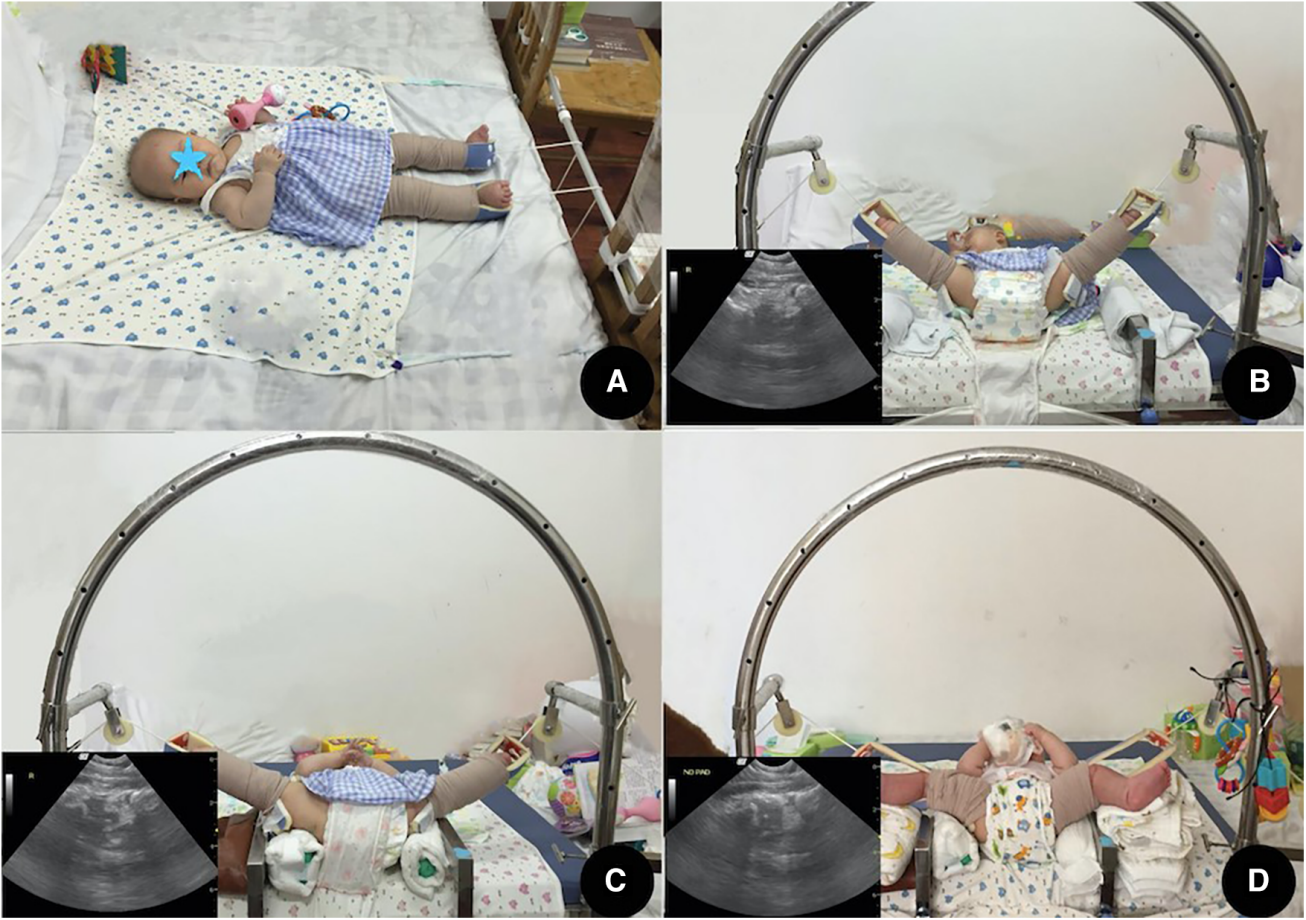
Two-stage traction. Stage 1: (A) horizontal traction at home. Stage 2: (B–D) ultrasound-guided vertical traction in the hospital. (B) Day 4, abduction was increased to 50°, and anterior hip ultrasound viewed the posterior dislocated femoral head. (C) Day 7, after using padding and adding the traction load, the femoral head was reduced to the acetabulum, but some distance between the femoral head and the acetabulum can be seen on the anterior hip ultrasound. (D) Day 12, after reducing the traction load, the femoral head gradually “docked” to a concentric position. The minimized distance between the femoral head and the acetabulum can be seen on the anterior hip ultrasound).
